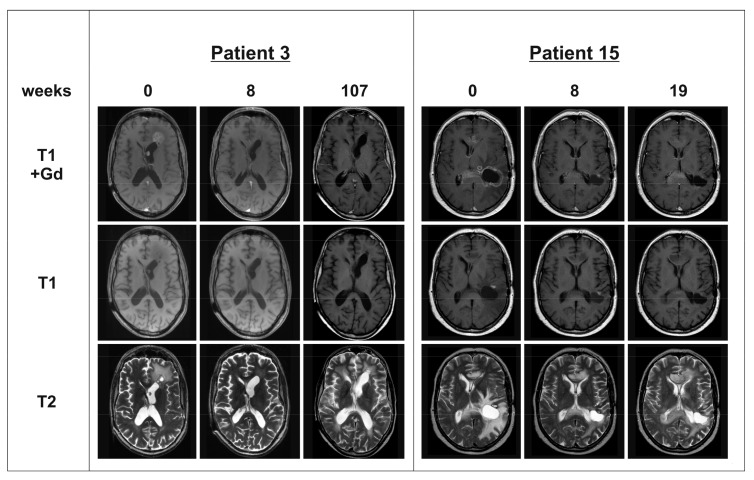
Magnetic resonance imaging (MRI) of patients 3 and 15: T1 sequences with and without Gadolinium (Gd) contrast enhancer and T2 sequences were obtained at baseline, follow-up at 8 weeks after Bevacizumab (BEV) therapy initiation and at relapse. Patient 3 achieved partial response (PR) under BEV therapy combined with irinotecan. At week 107, patient 3 showed a progressive contrast enhancement in the area of the septum pellucidum. Patient 15 reached PR under BEV monotherapy. At week 19, both contrast-enhancing lesions (in the anterior and posterior part of the corpus callosum) progressed.
